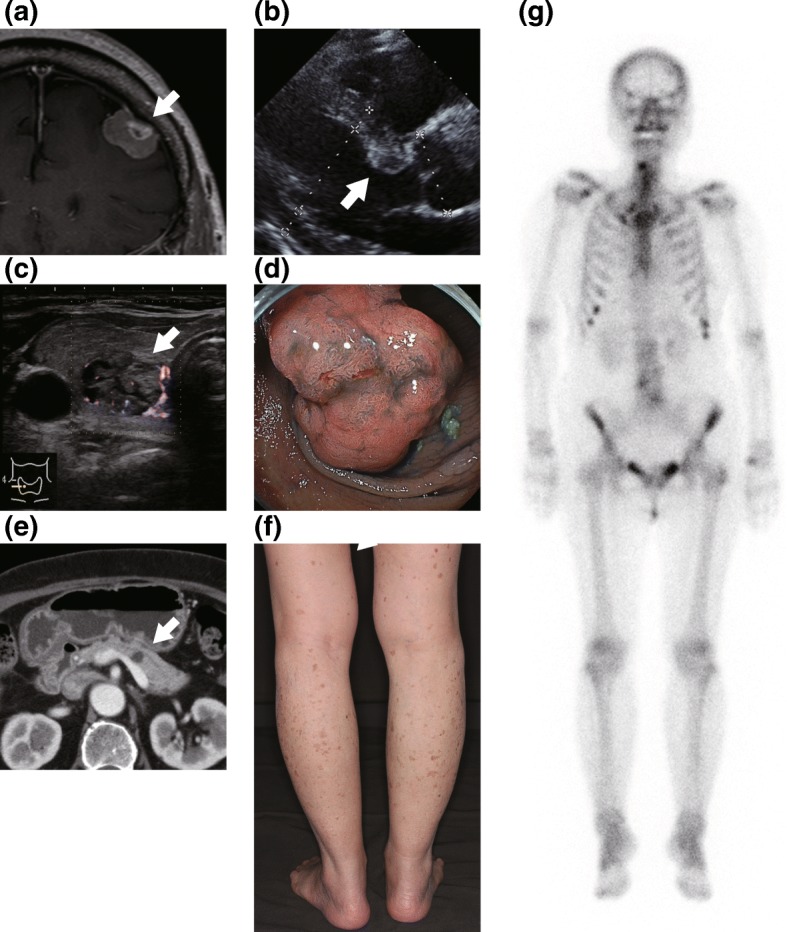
Imaging studies of extra-adrenal tumors in this patient. a A convexity meningioma 21 × 13 × 18 mm in size on enhanced T1WI MRI of the Head (arrow). The pituitary tumor is not marked. b A hyperechoic lesion about 14 mm in size in the interventricular septum on echocardiography (arrow). c Cervical ultra-sonography revealed four tumors in both thyroid lobes (arrow). d Colonoscopy shows tubular adenocarcinoma in the colon. This tumor was later resected, but the status of *GNAS* mutation was not assessed. e Enhanced abdominal computed tomography showed cystic lesions compatible with intraductal papillary mucinous neoplasm in the pancreatic body (arrow). f Brown patchy pigmentations were observed systemically. Skin appearance with patchy pigmentations of bilateral lower limbs is shown. g X-ray imaging and bone scintigraphy with ^99m^Tc-scintigraphy did not indicate fibrous dysplasia
